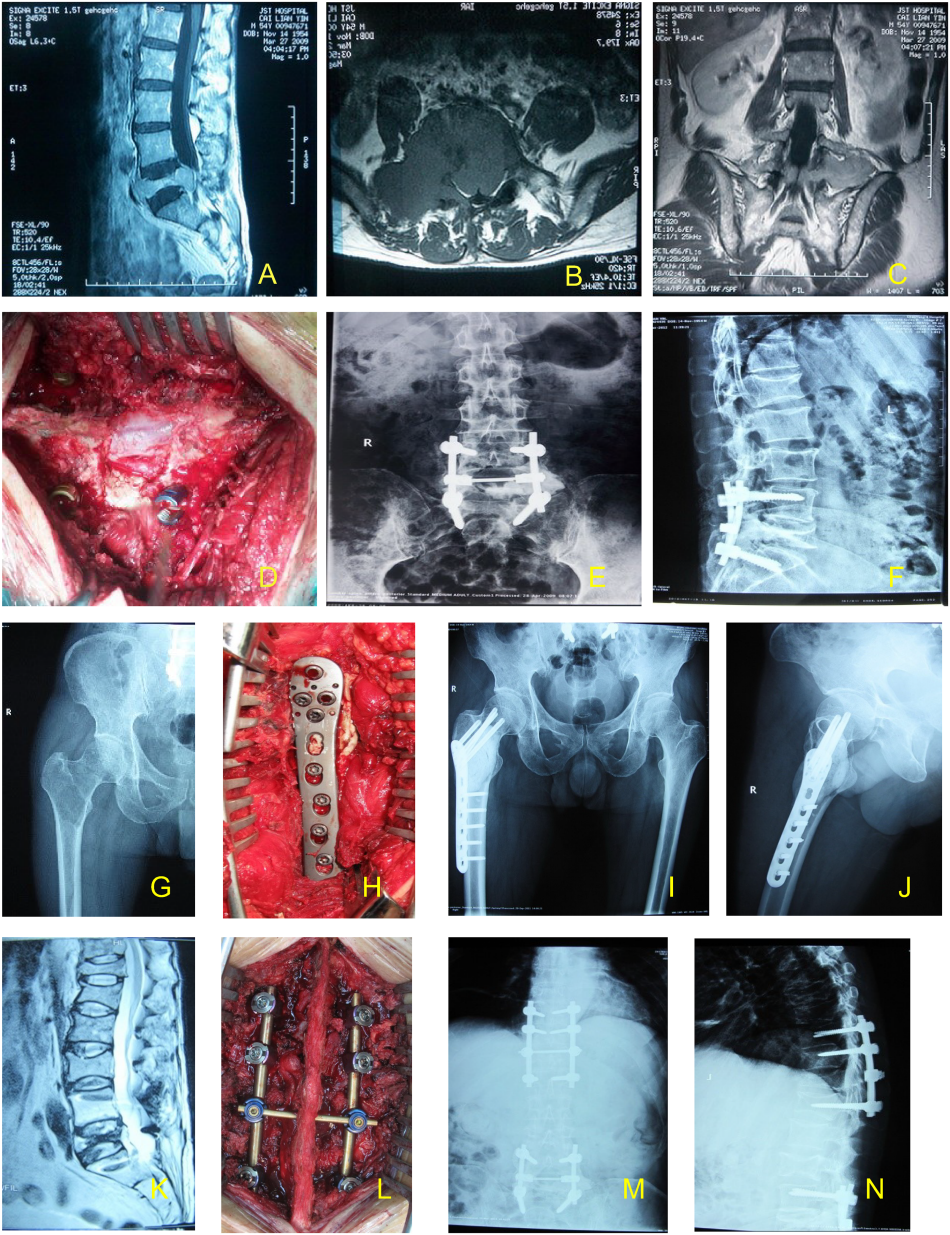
The case of a patient undergoing triple sugery. The first surgery is represented by (A–F). (A–C) Prior to surgery, the lesion was located using high-resolution magnetic resonance imaging. (D) Surgical field during the first surgery. (E,F) Following surgery, the lesion was located by high-resolution magnetic resonance imaging. The second surgery is represented by (G–J). (G) MRI of patient prior to the second surgery. (H) Surgical field during the second surgery. (I,J) Following the second surgery, the lesion was located by high-resolution magnetic resonance imaging. The third surgery is represented by (K–N). (K) MRI of patient prior to the third surgery. (L) Surgical field during the third surgery. (M,N) Following the third surgery, the lesion was located by high-resolution magnetic resonance imaging. MRI, magnetic resonance imaging.
